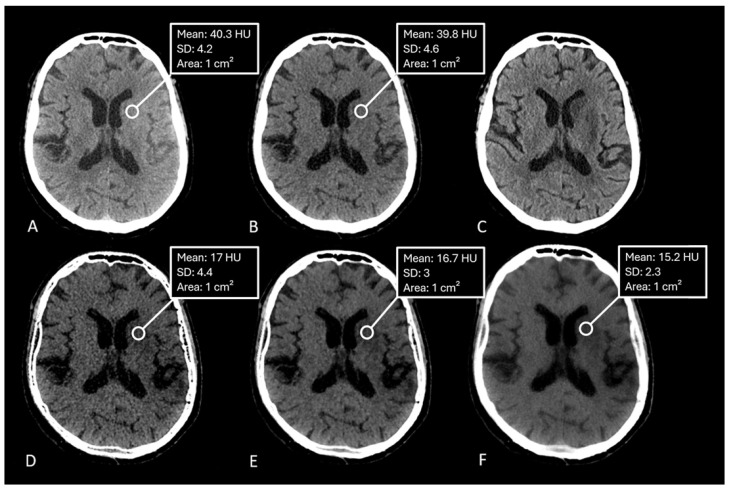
CT-Images of a 71 year old male after recanalization of a M1-occlusion on the left. (A): Mixed images with no visible infarction of the basal ganglia, (B): conventional virtual non-contrast map with barely visible infarction, (C): follow-up CT after 5 days with Infarction of the basal ganglia, (D): edema map at smoothing level 1 with visible infarction of the basal ganglia, (E): edema map at smoothing level 3 with clearly visible infarction of the basal ganglia and good image quality, (F): edema map at smoothing level 5 with clearly visible infarction of the basal ganglia.
